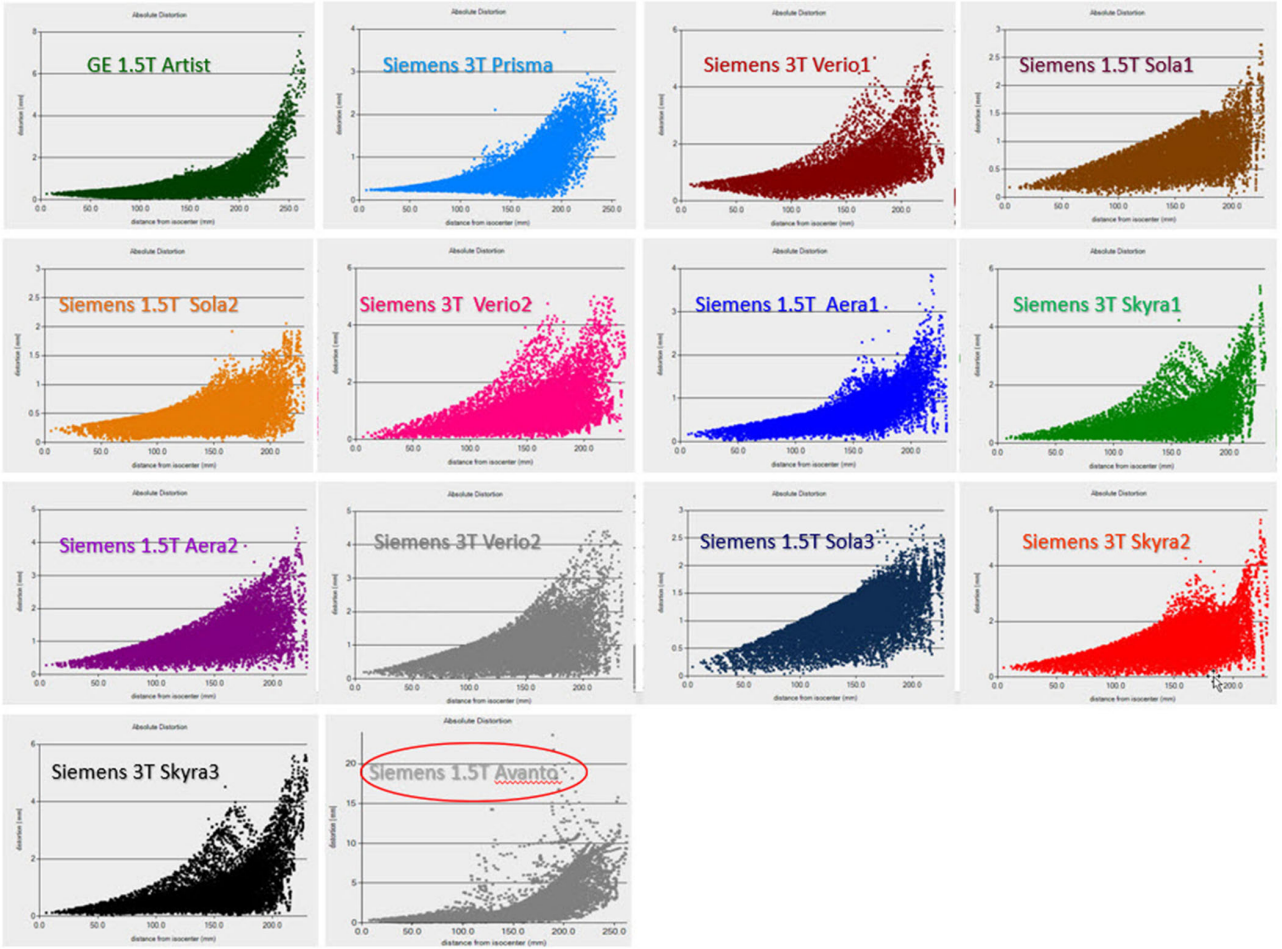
The magnitude of MRI image distortion versus the distance from the imaging isocenter for all the 14 scanners studied
